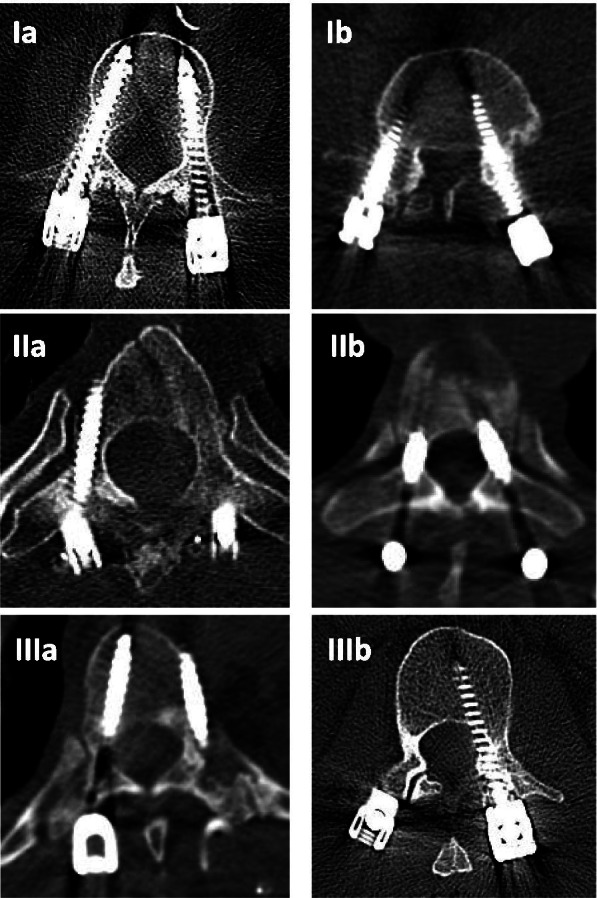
Exemplary CT scans showing the different grades of Zdichavsky classification
